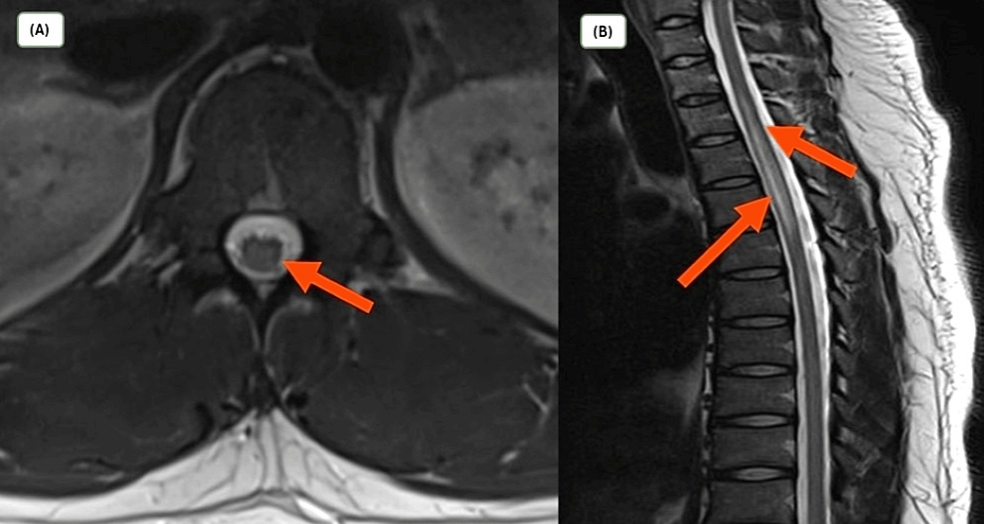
T-2 weighted spinal MRI demonstrating hyperintense signals from the T2-T6 vertebrae (A, B)
